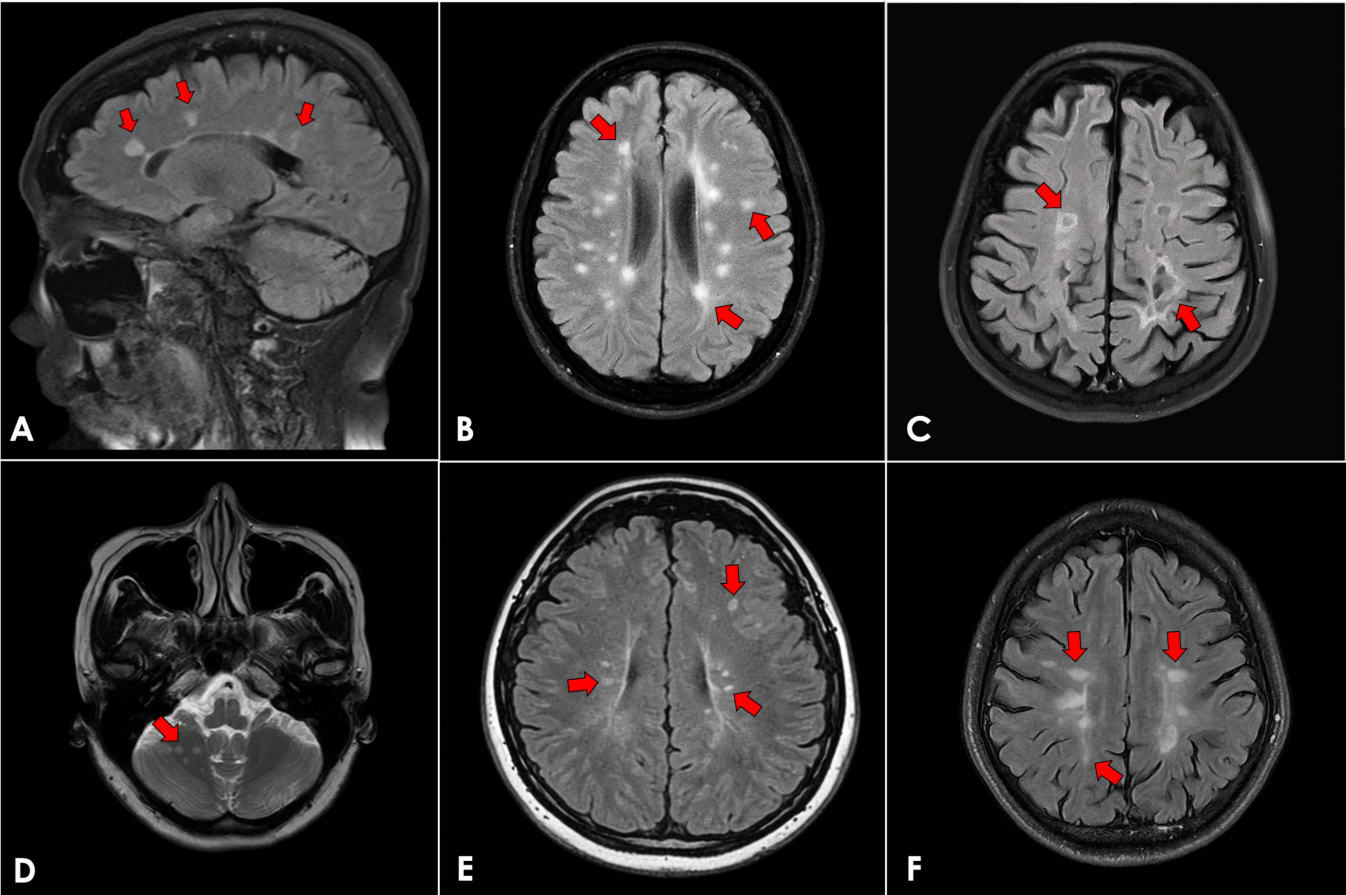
Representative pre-aHSCT brain MRI images. (A) Case 1: sagittal FLAIR image demonstrating periventricular hyperintense lesions (arrows). (B) Case 2: axial FLAIR image showing hyperintense lesions in the periventricular, juxtacortical, and subcortical regions (arrows). (C) Case 3: axial FLAIR image demonstrating periventricular and subcortical hyperintense lesions (arrows). (D) Case 4: axial T2-weighted image showing hyperintense lesions in the cerebellar hemisphere (arrow). (E) Case 5: axial FLAIR image demonstrating juxtacortical and periventricular hyperintense lesions (arrows). (F) Case 6: axial FLAIR image showing juxtacortical and subcortical hyperintense lesions (arrows) Abbreviations: aHSCT = autologous hematopoietic stem cell transplantation; MRI = magnetic resonance imaging; FLAIR = fluid-attenuated inversion recovery
